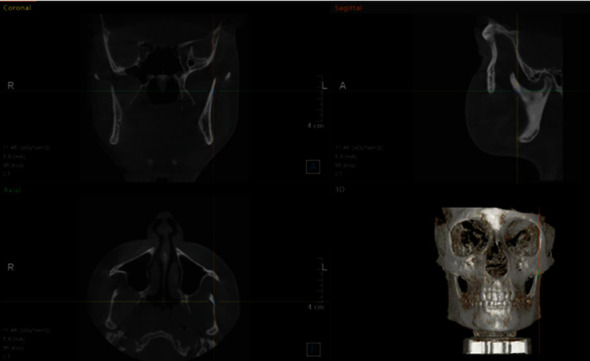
Standardization CBCT image for condyle-glenoid fossa measurements.
